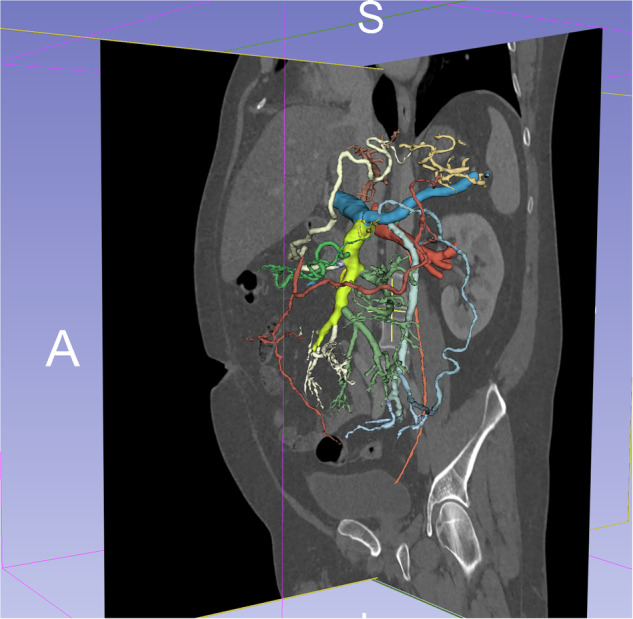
Sagittal and coronal CT overlays of the venous map (colors as in Fig. 3) for clearer spatial context; large vessels may be semi-automated, whereas segments lesser than 4 mm typically require manual delineation.
